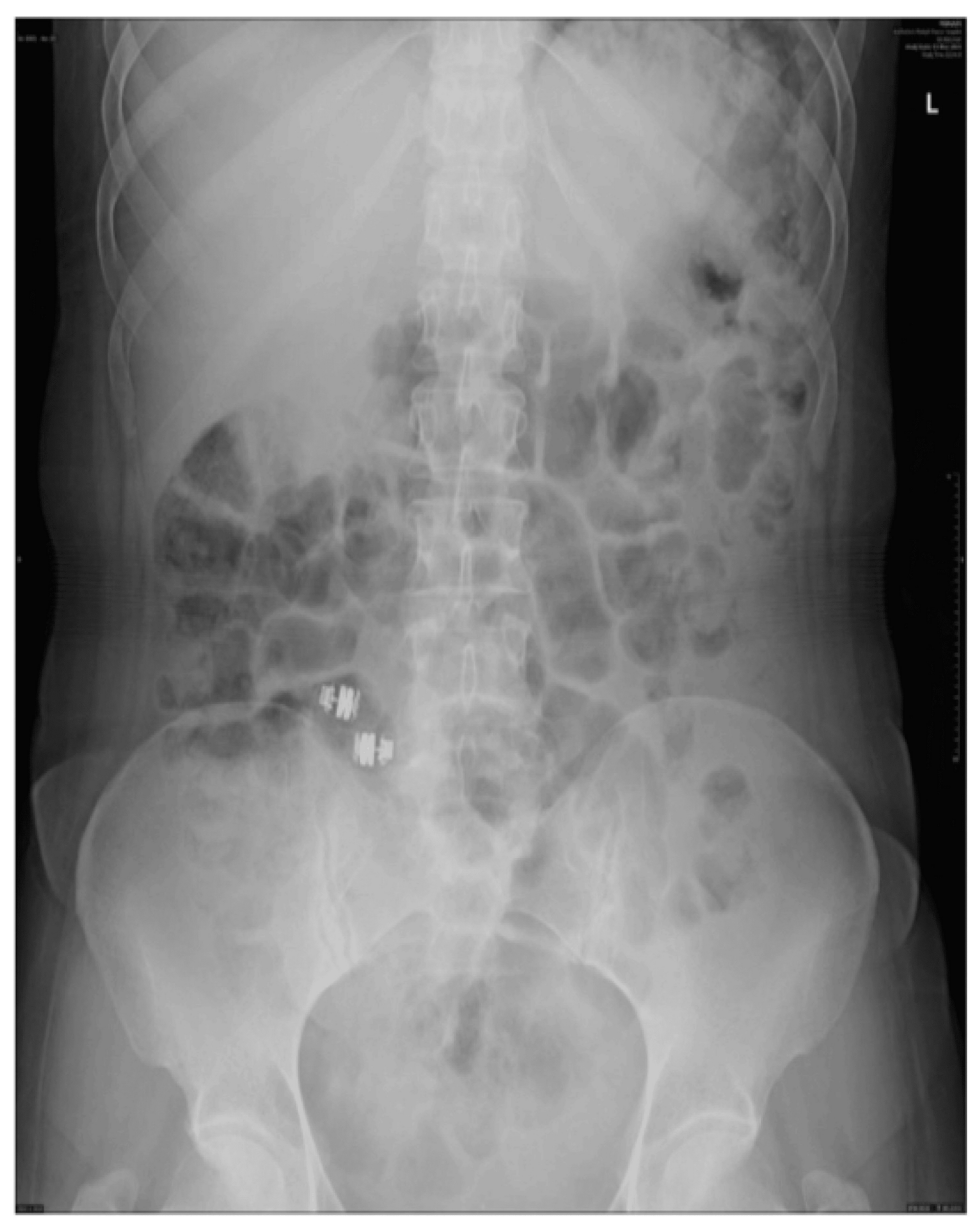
Abdominal X-ray showing two capsules retained at the terminal ileum
